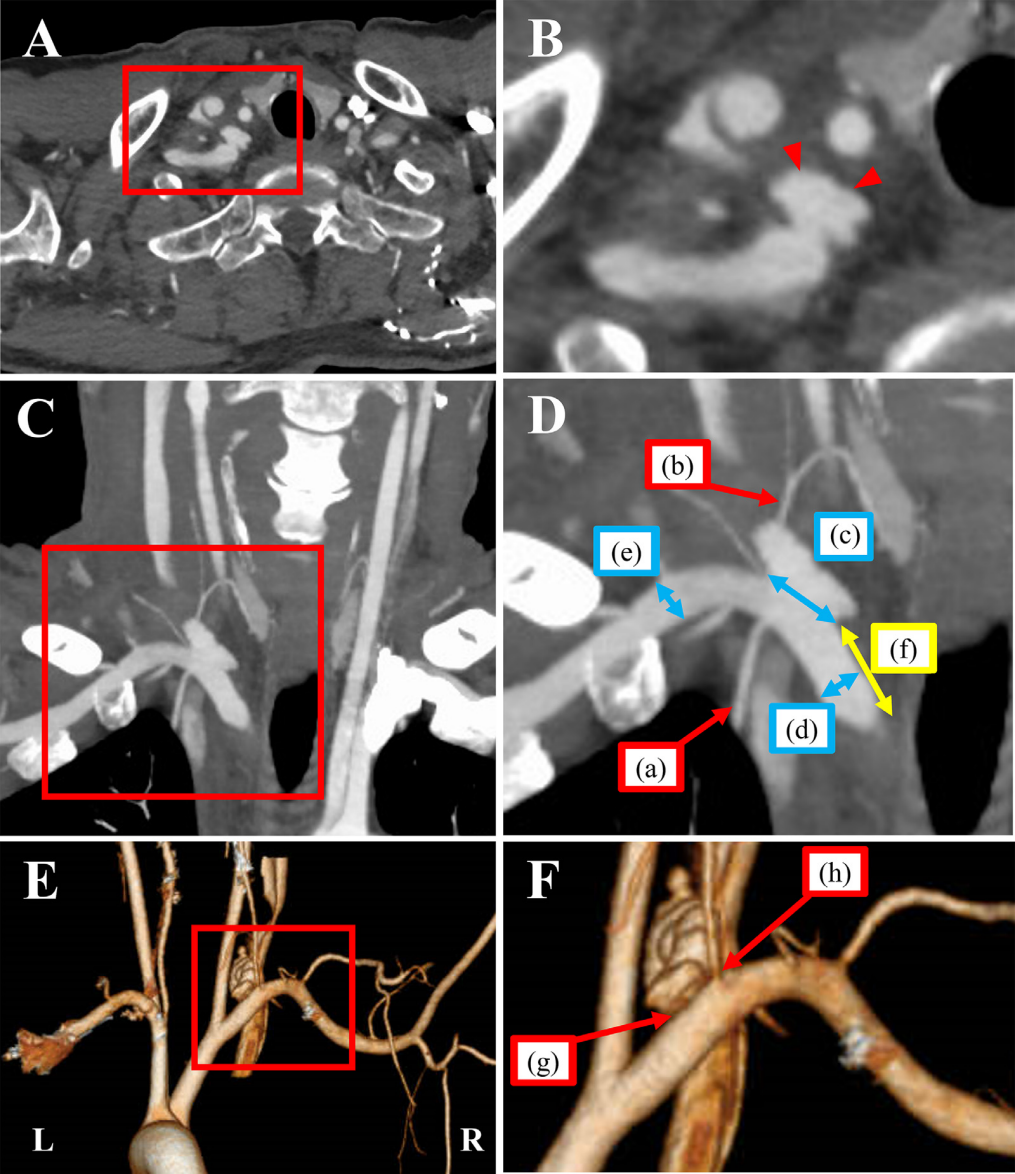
Contrast-enhanced computed tomography (CT) scan taken by the previous physician. Contrast-enhanced CT findings, revealing (A, B) a large saccular pseudoaneurysm (red arrow). (C, D) The pseudoaneurysm, located 13.0 mm from the right subclavian artery bifurcation, had an inlet diameter of 3.0 mm. The proximal and distal diameters of the pseudoaneurysm were 8.0 mm and 6.0 mm, respectively (a: right internal thoracic artery, b: right thyrocervical artery, c: 3.0 mm, d: 8.0 mm, e: 6.0 mm, f: 13.0 mm). (E, F) A dorsal view of the 3D reconstruction of the contrast-enhanced CT image showed the proximity of the pseudoaneurysm and the right vertebral artery (G: pseudoaneurysm, H: right vertebral artery).
